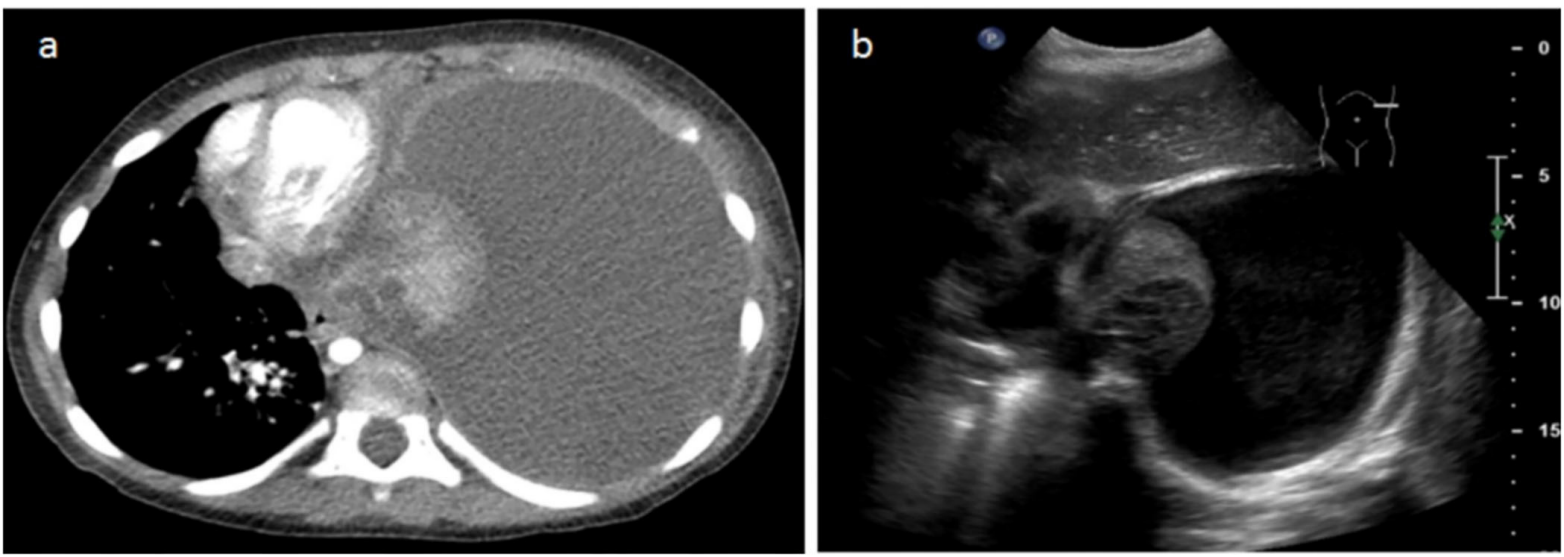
A 3-year-old female patient with severe dyspnea. (a) The transverse slice chest CT demonstrates massive left effusion and atelectasis of the entire left pulmonary parenchyma, with heterogeneous enhancement and round necrotic unenhanced lesions posteriorly. (b) LUS performed the same day demonstrates the same parenchymatous damage with coalescent cystic lesions corresponding to necrosis.
